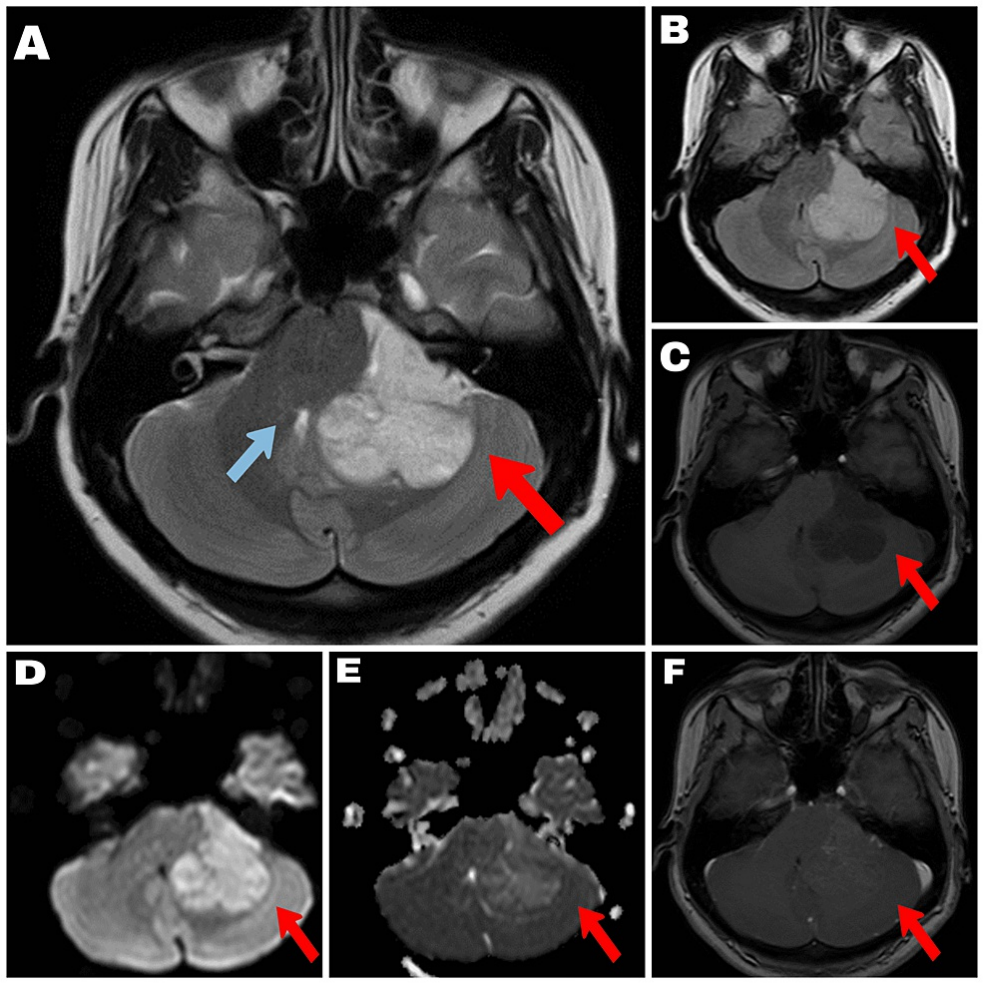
MRI characteristics of the lesion A 4cm x 4.5cm extra-axial space occupying lesion in the left cerebellopontine angle cistern, compressing the left cerebellar hemisphere , medulla, pons and mid brain. The lesion is not causing internal auditory meatus widening/extension, no white matter edema and has the following MRI characteristics: A- T2: Homogenous hyperintense; B- FLAIR: Homogenous hyperintense; C- T1: Homogenous hypointense; D- DWI: Homogenous hyperintense; E- ADC: Homogenous isointense; F- T1 contrast: Minimal heterogenous enhancement with few cystic areas. Red arrow demonstrates the lesion in all sequences, blue arrow in sequence A depicts brain stem distortion with compression of the fourth ventricle.
